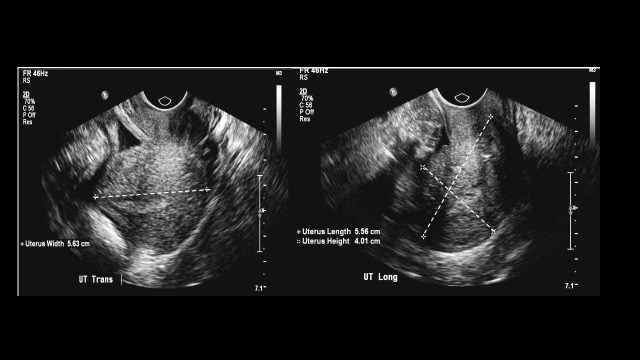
Ultrasound of the abdomen shows a large intrauterine mass.
